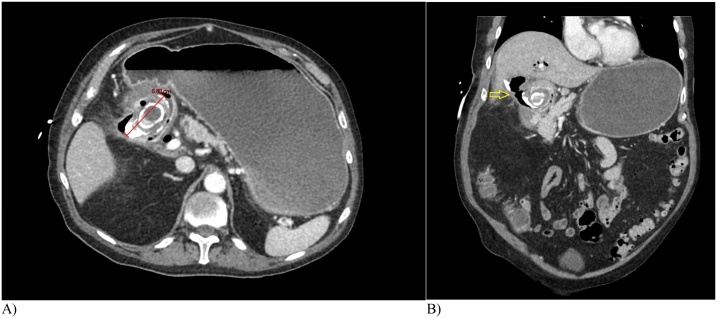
Computed Tomography Abdomen Axial and Coronal. A) 5.8 cm (red line) laminated and partially calcified stone is noted in the first and second portions of the duodenum with B) pneumobilia suggesting cholecysto-duodenal fistula (yellow arrow) and associated signs of gastric outlet obstruction.
